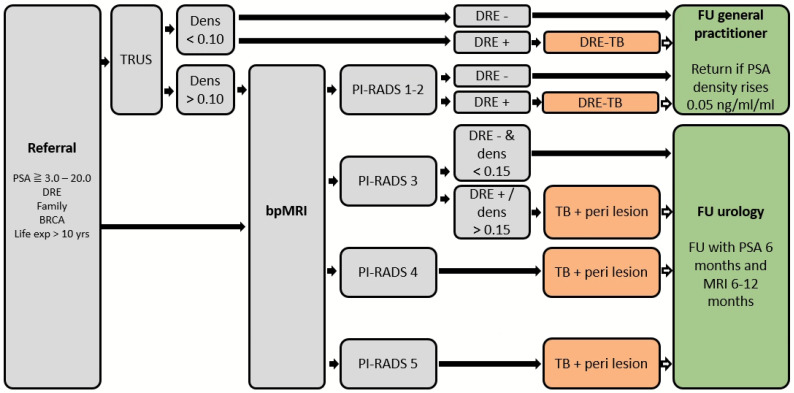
Example of a risk-based pathway for suspected prostate cancer, MRI-guided and using targeted biopsies only. BRCA—breast cancer (gene mutation); Bp—biparametric; Dens—density; DRE—digital rectal examination; Exp—expectancy; FU—follow-up; MRI—magnetic resonance-imaging; PIRADS—prostate imaging reporting and data system; PSA—prostate-specific antigen; PSMA—prostate-specific membrane antigen; TB—targeted biopsy; TRUS—transrectal ultrasound.
